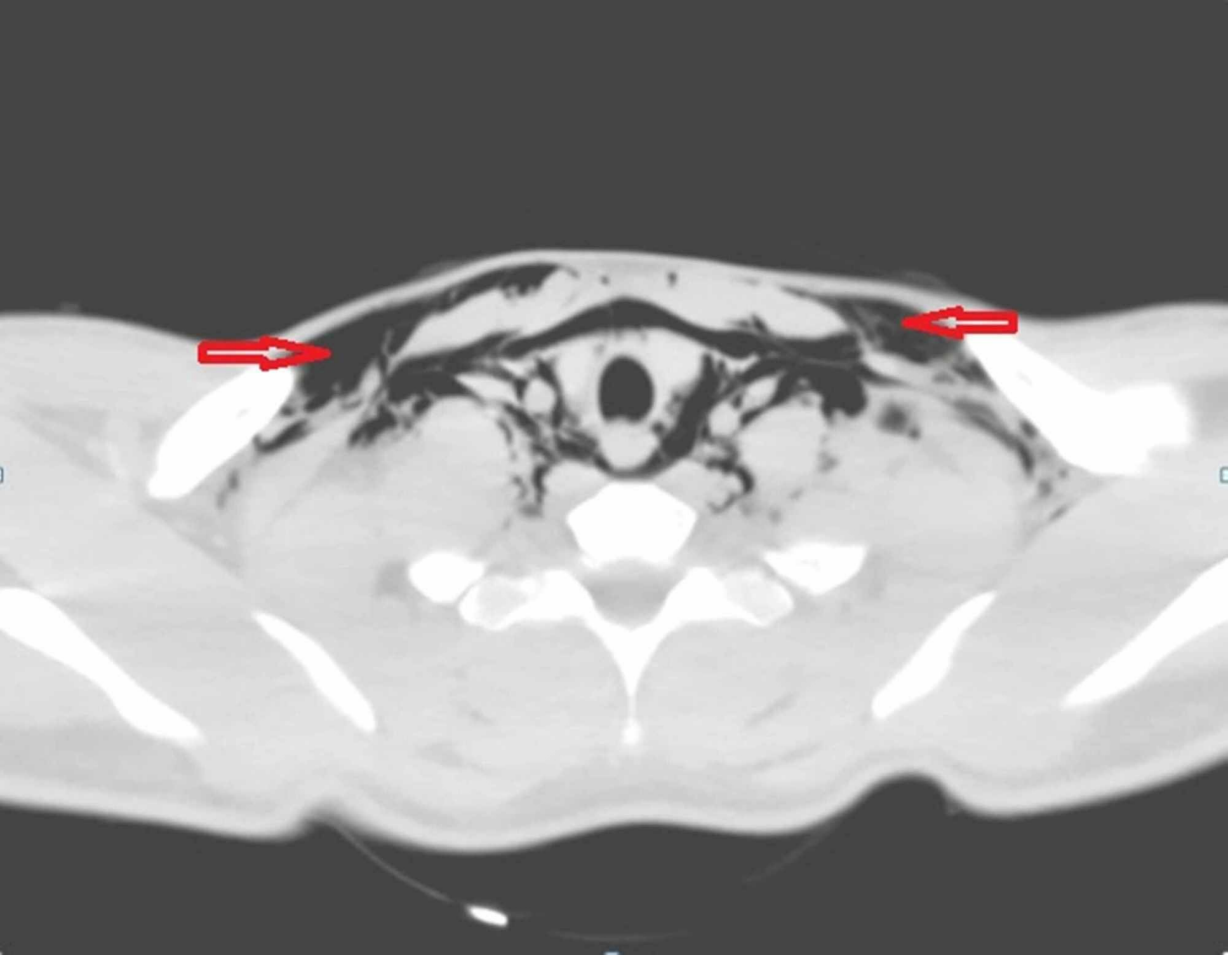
Non-contrast computed tomography (NCCT) of the neck showing subcutaneous emphysema (arrows)
